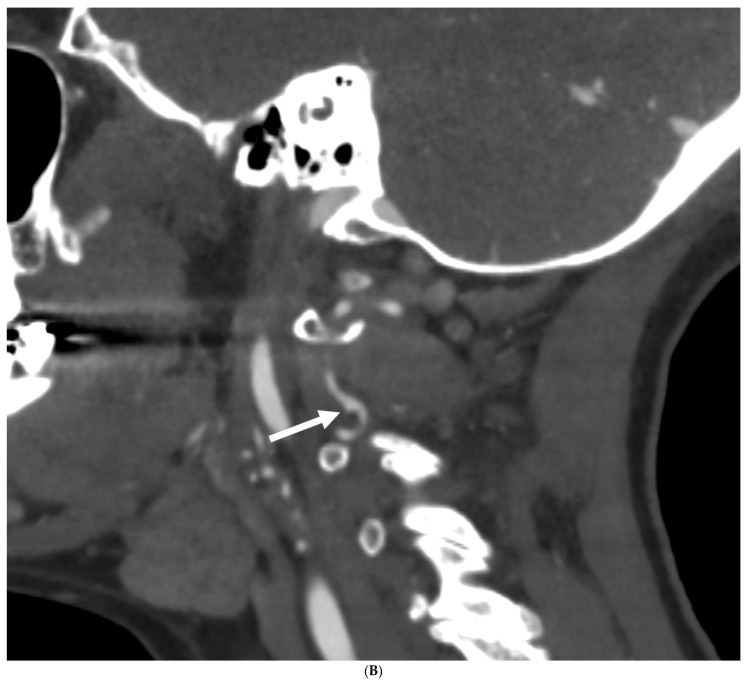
(A) 67-year-old woman with hEDS and a history of chronic migraine. Axial CT angiography image demonstrates a laterally projecting pseudoaneurysm of the left mid cervical internal carotid artery measuring up to 2.6 mm in depth (arrow), a sequelae of prior dissection. (B) 60-year-old woman with HSD and a history of episodic migraine. Sagittal CTA image demonstrates a tiny focal outpouching of the V3 segment of the left vertebral artery (arrow) compatible with a tiny pseudoaneurysm, a sequelae of prior dissection.
